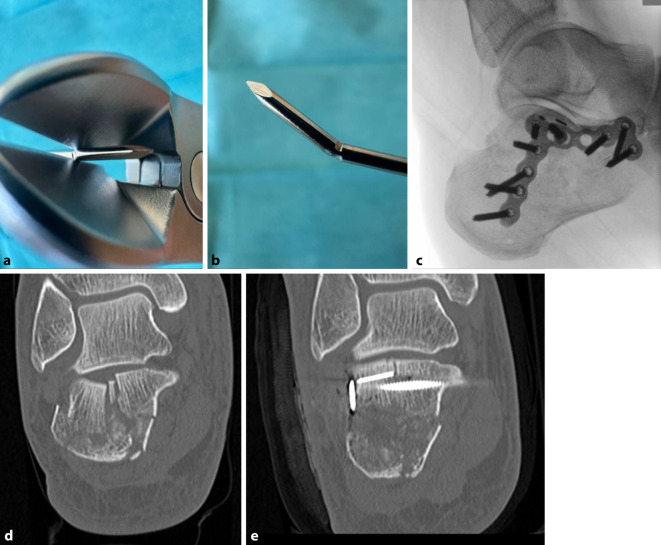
Beispielhafte Darstellung der „Lost-wire“-Anwendung mit a Präparation der Sollbruchstelle mittels Seitenschneider und b gezieltem Abbrechen der Drahtspitze nach Einbringung; c intraoperatives laterales BV-Bild mit „lost wires“ unterhalb der Gelenkfläche, d prä- und e postoperative koronare CT-Schnittbildebene
